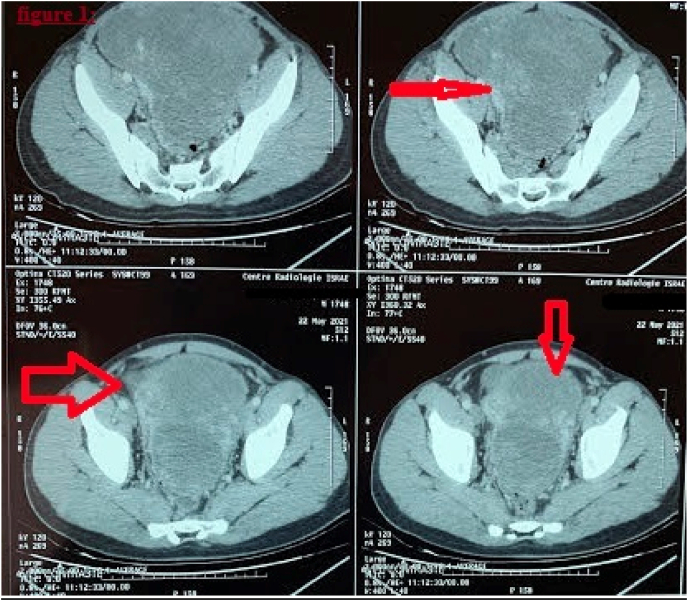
Enhanced Abdomino-pelvic computed tomography demonstrates a voluminous mass centered on the retro-bladder region (arrow).
